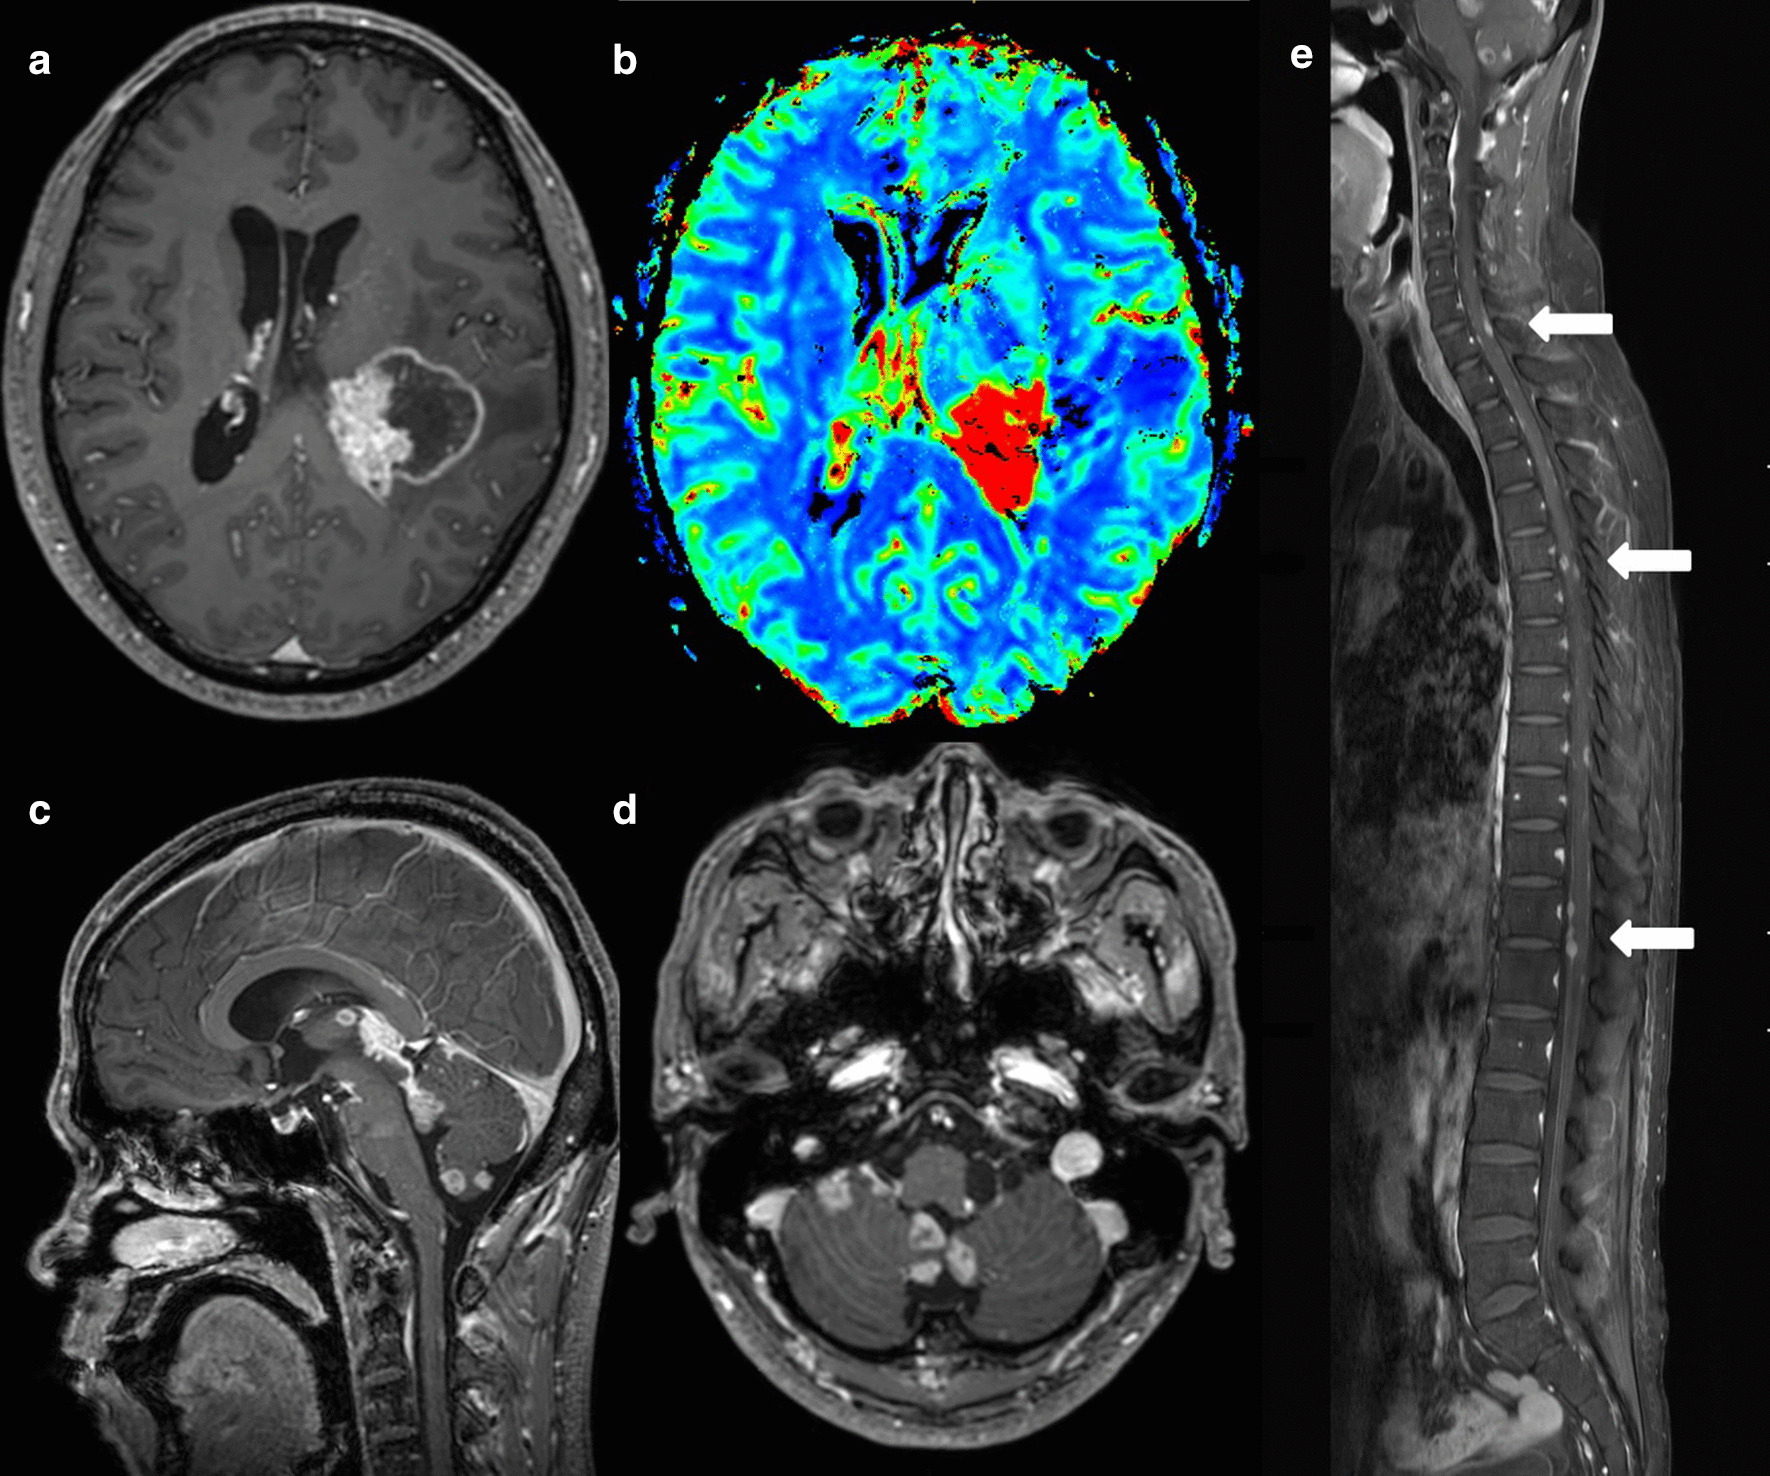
Preoperative magnetic resonance imaging scans (a–e). Brain MRI with gadolinium enhancement revealing heterogeneous enhancing masses in the left thalamus, left parietotemporal lobe, posterior part of left lateral ventricle, left temporal horn, interpeduncular cistern, quadrigeminal cistern, and right cerebellopontine angle cistern. a, c, d Perfusion MRI showing increase in cerebral blood flow at the mass lesion. b Spine MRI revealing multiple linear and nodular contrast-enhancing lesions (white arrows) within the cord and leptomeninges in the entire spine area (e)
